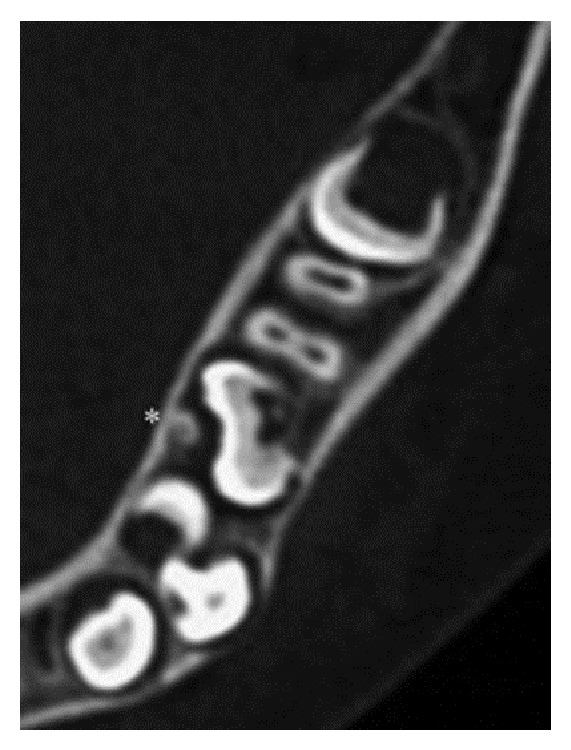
Axial computed tomography image: case  1. A small calcium deposit (∗) located lingual to the mandibular right second primary molar.
